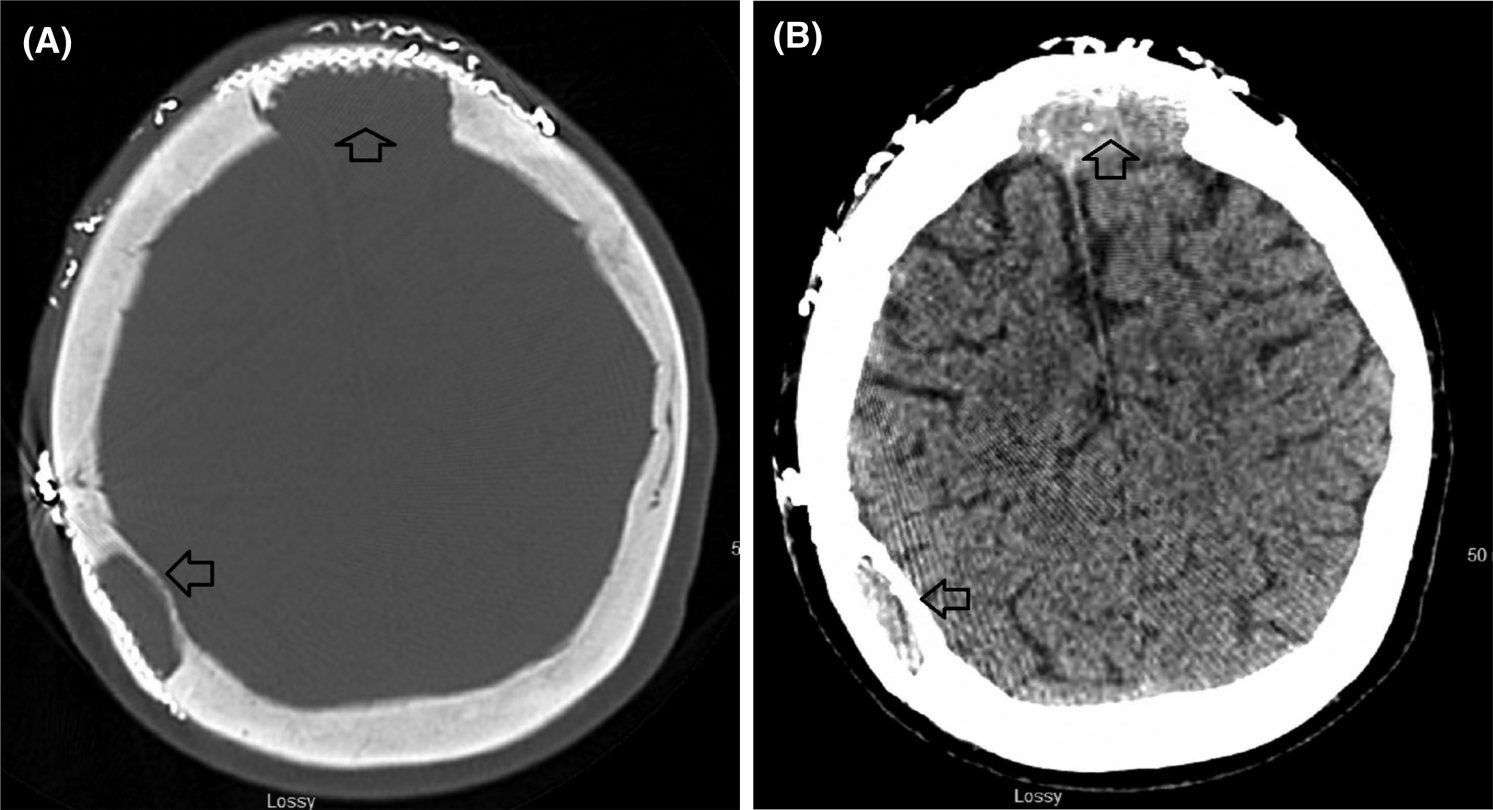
(A) Head CT scan without contrast axial view (bone‐window) at 6‐month postoperative follow‐up visit revealing an intact Titanium mesh implants around the site of bony resections. (B) Head CT scan without contrast axial view at 6‐month postoperative follow‐up visit revealing a gross total resection of all calvarial, and extracalvarial lesions.
